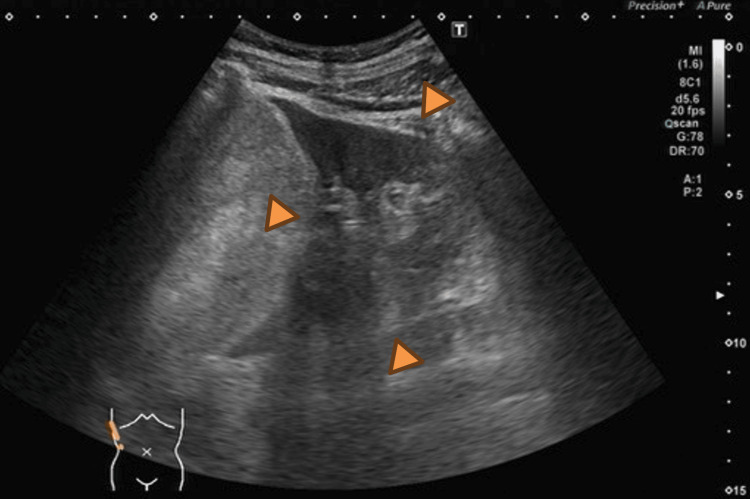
Abdominal ultrasonography findings Ultrasonography demonstrates a heterogeneous mass with mixed echogenic components and partially hyperechoic areas
